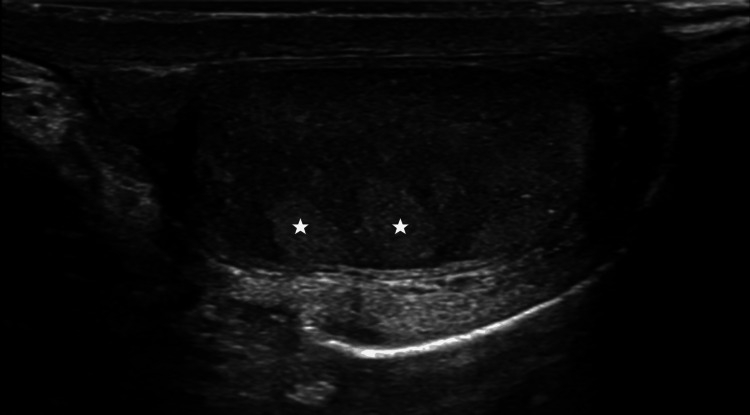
Ultrasonography of the testis showing multiple non-shadowing calcific hyperechogenic foci of varying sizes (of which two have been marked with white stars), suggestive of testicular microlithiasis.
